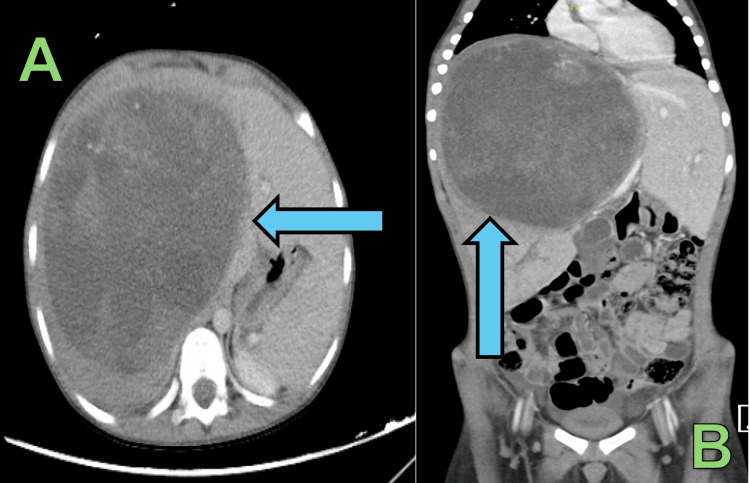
CT abdomen with IV contrast showing hypervascular lesion in the right lobe measuring 15.8 × 12.8 × 16 cm A: Axial view; B: Coronal view
